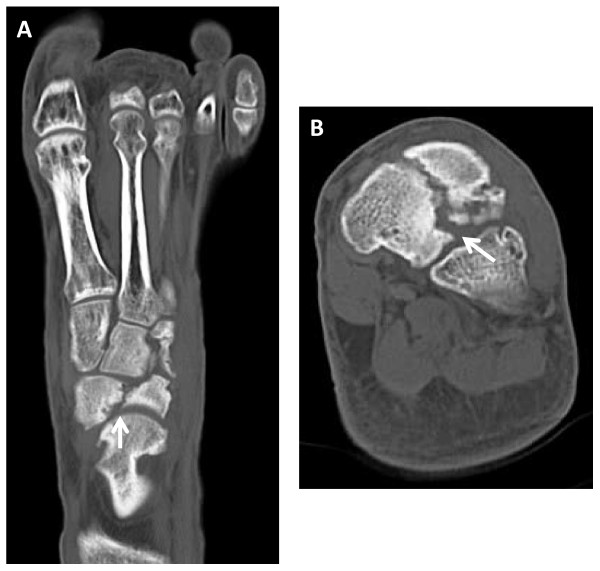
Computed tomography scans at the time of presentation. (A) Axial image. (B) Coronal image.
